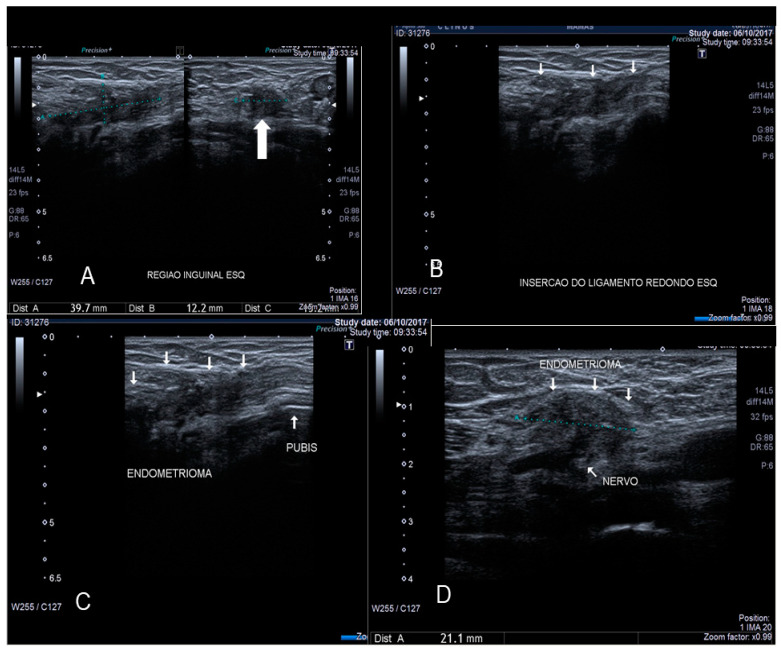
Sonographic features of solid inguinal endometriosis (see thin and thick with arrows) (A–D).
